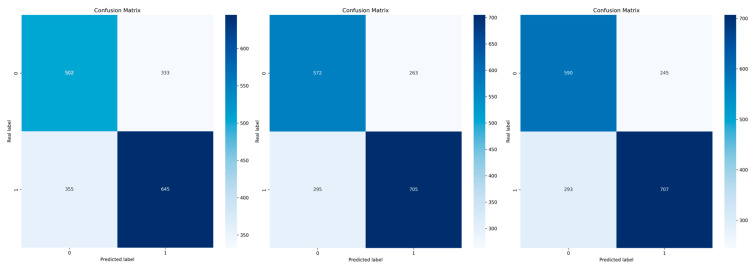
Confusion matrices of the progression and radionecrosis categories indicating true and predicted observations for Logistic Regression (left), multilayer perceptron (MLP) (middle), and AdaBoost (right). The total number of categories corresponds to the total number of patients in the training set multiplied by the number of temporal DSC MRI sequences.
